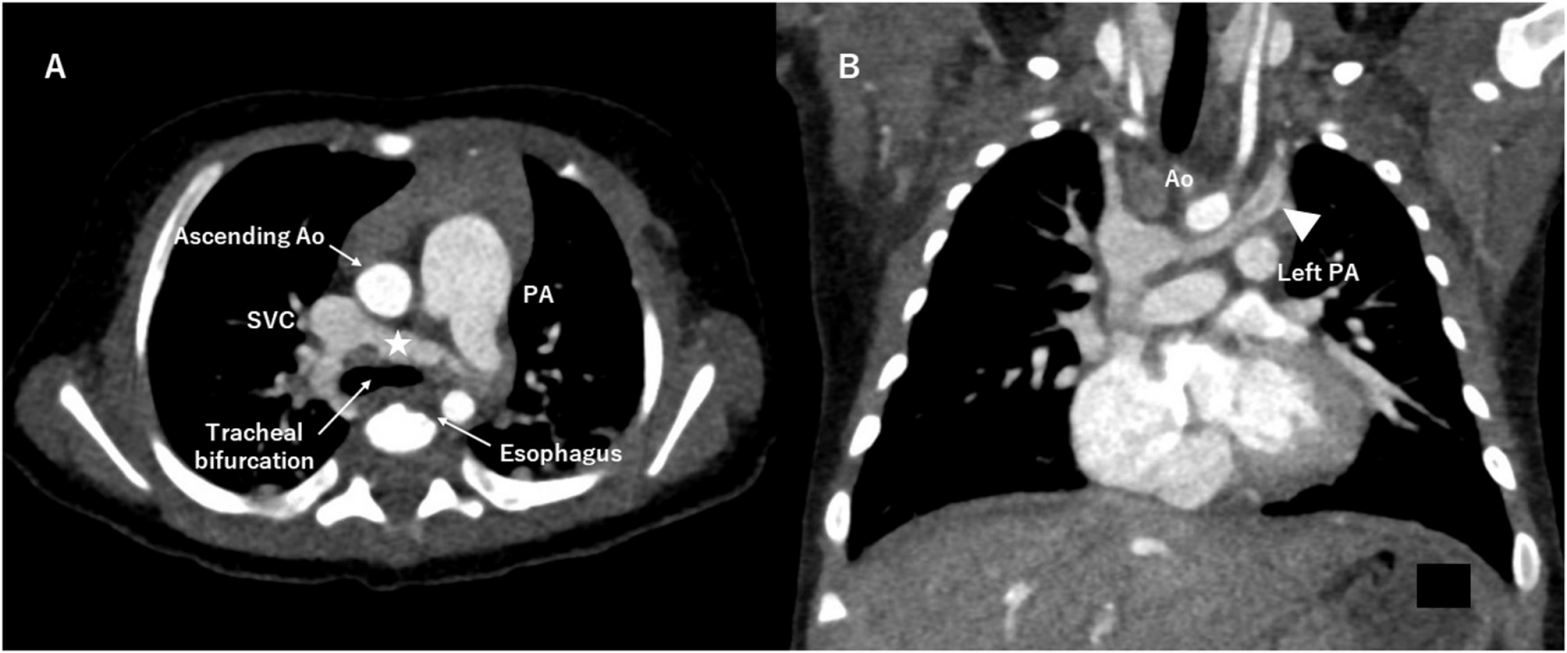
Computed tomography angiography. (A) The retroaortic innominate vein (RAIV, asterisk) runs near the tracheal bifurcation and the esophagus. (B) The descending portion of the RAIV (arrowhead) is similar to a persistent left superior vena cava. Ao indicates aorta; PA, pulmonary artery; SVC, superior vena cava.
